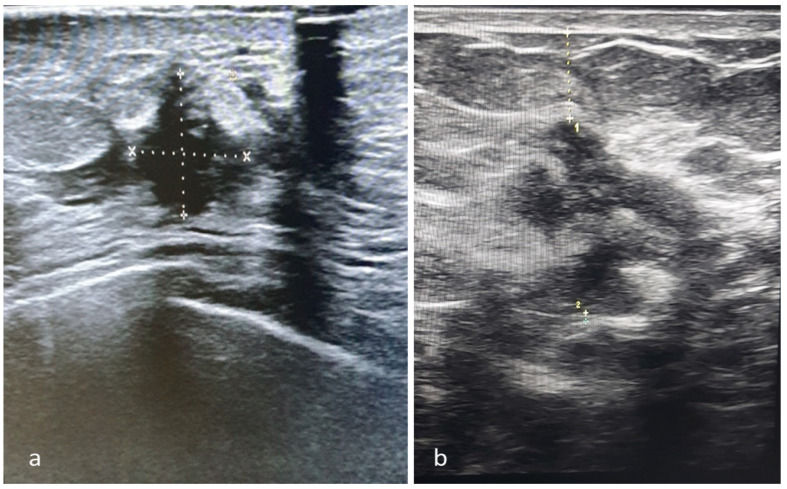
The ultrasound measurements. (a) Largest horizontal (craniocaudal and mediolateral) and vertical diameters of the tumor. (b) Distances of the superficial margin of the tumor–skin (1) and the deepest margin–pectoral fascia (2).
